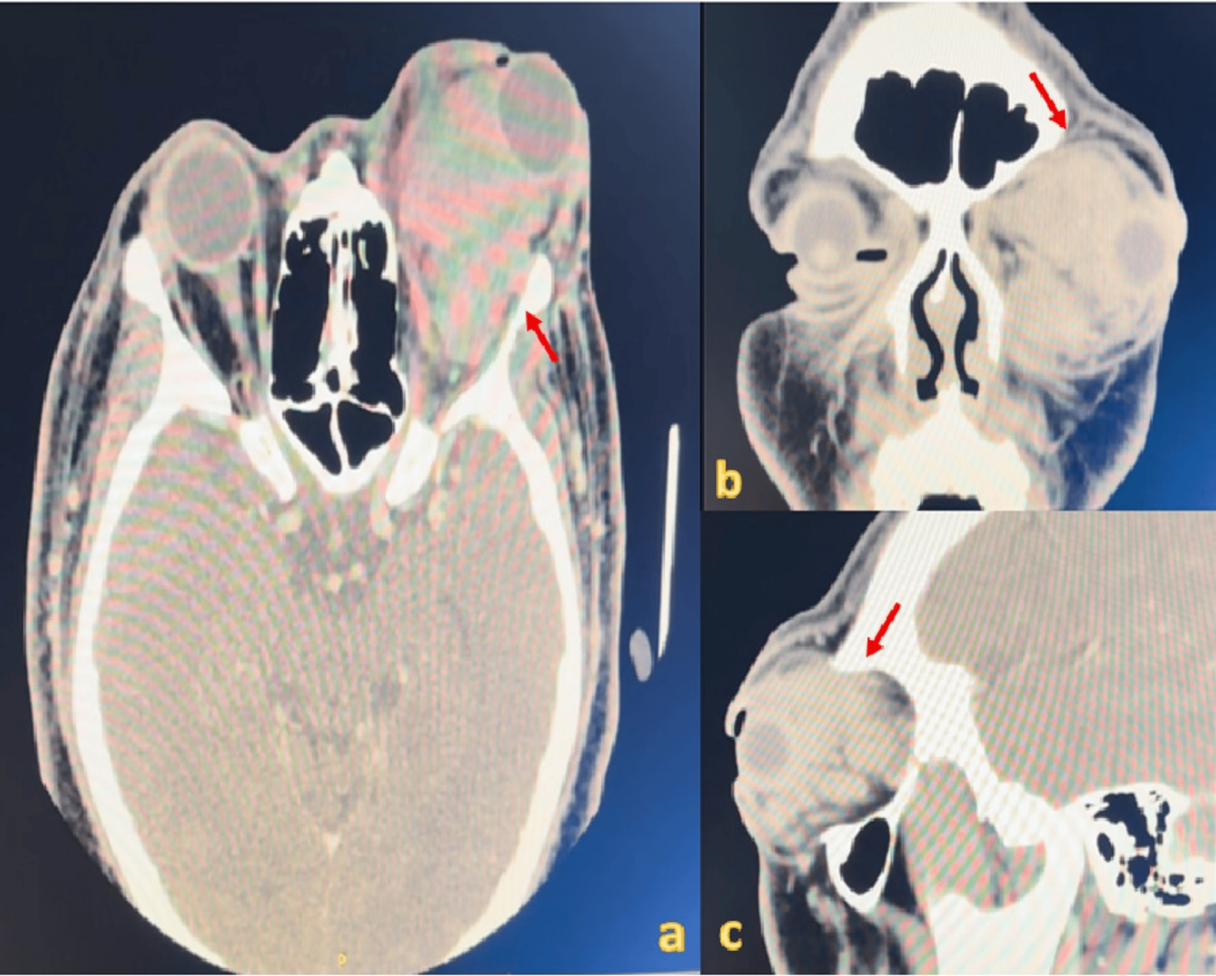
Computed tomography (CT) of the brain and orbit a. CT in axial cut; b. CT in coronal cut; c. CT in sagittal cut. The red arrow shows a large, multilobulated intraorbital soft tissue mass.
